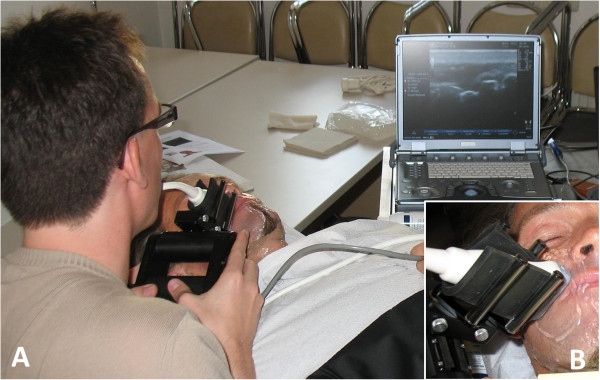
3D ultrasonographic examination of facial muscles. The linear array transducer was attached to a motorized 3D US acquisition system driven by a custom built linear mover connected to a laptop computer (A). Details of the attachment of the transducer to the 3D acquisition system are shown in (B).
